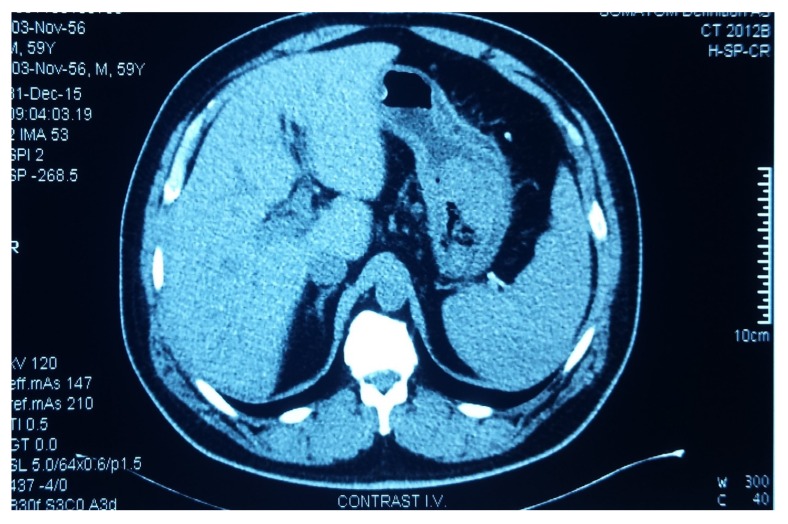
Computer Tomography (CT) scan showing the gastric tumor.
